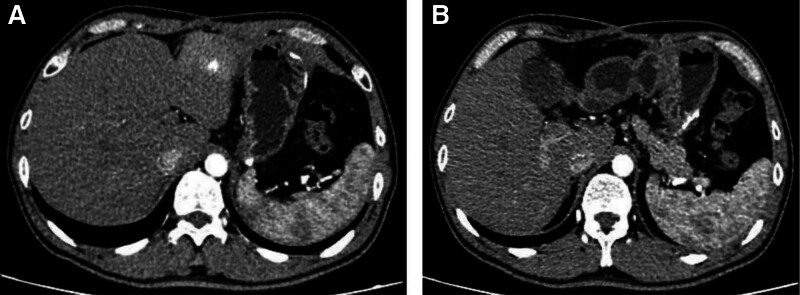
Enhanced CT scan results at the 2-month postoperative follow-up show no significant mass, with a clear outline of the pancreatic capsule. CT = computed tomography.
